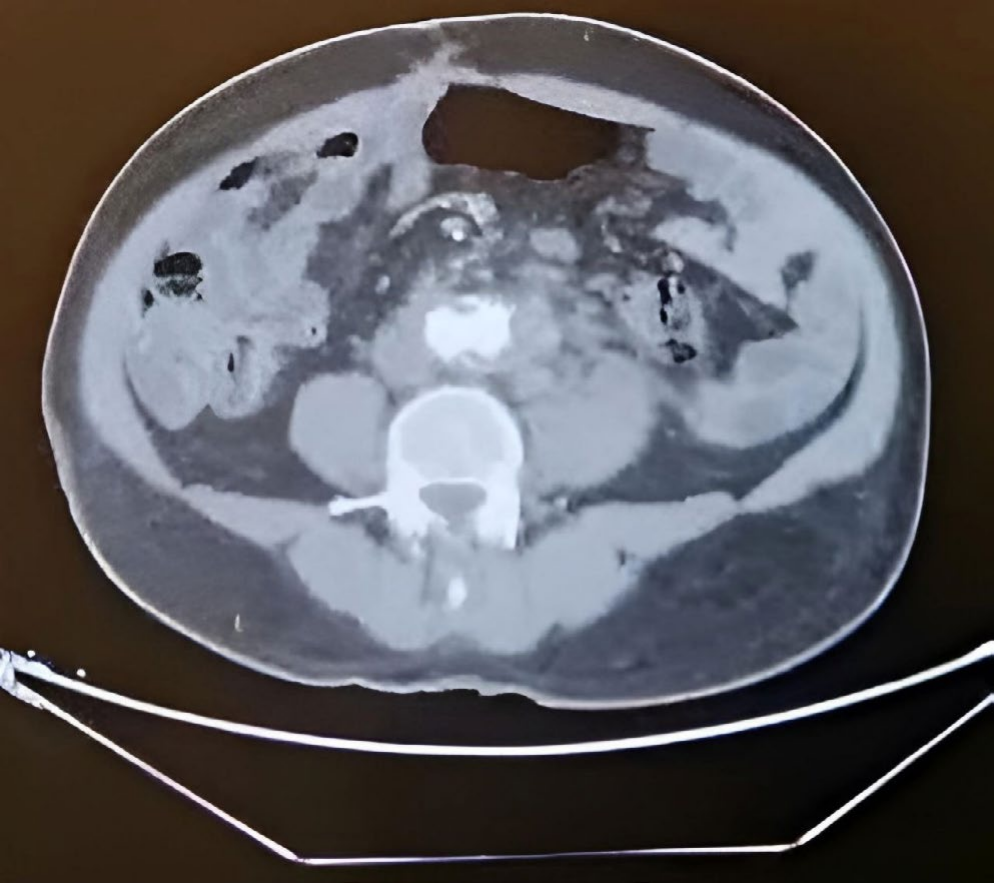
Axial contrast‐enhanced CT angiography (CTA) showing infrarenal abdominal aortic aneurysm with mural thrombus.
